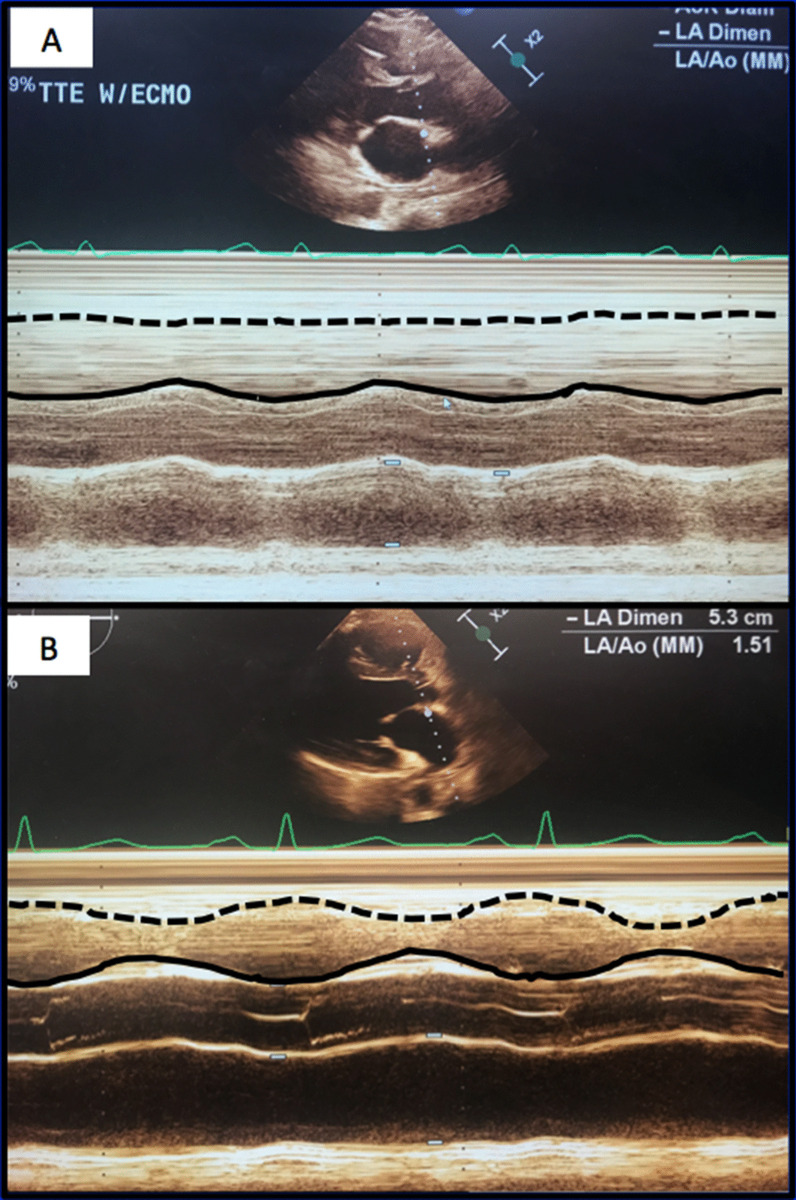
a M-mode transthoracic echocardiogram in parasternal long-axis demonstrating poor RV contractility in early postoperative period (a) and near normal RV contractility four months later (b). Dotted line represents RV free wall, solid line represents inter-ventricular septum
